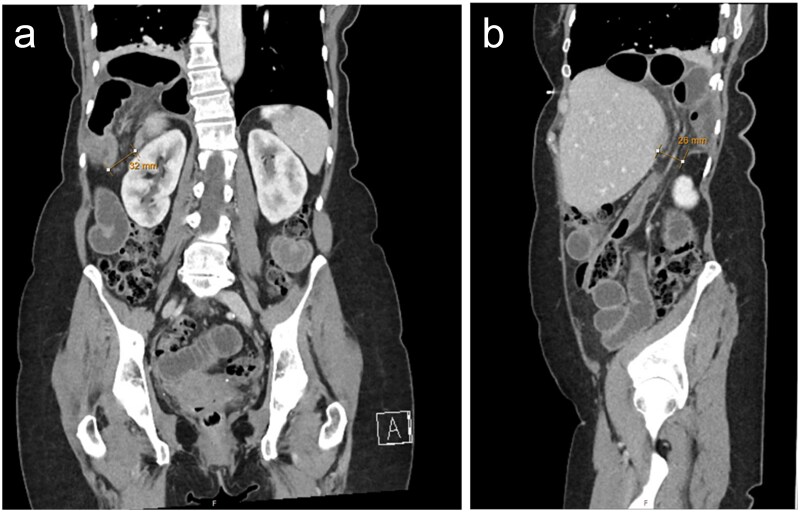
CT with a) coronal and b) sagittal slices demonstrating right sided posterolateral diaphragmatic defect containing incarcerated small bowel.
